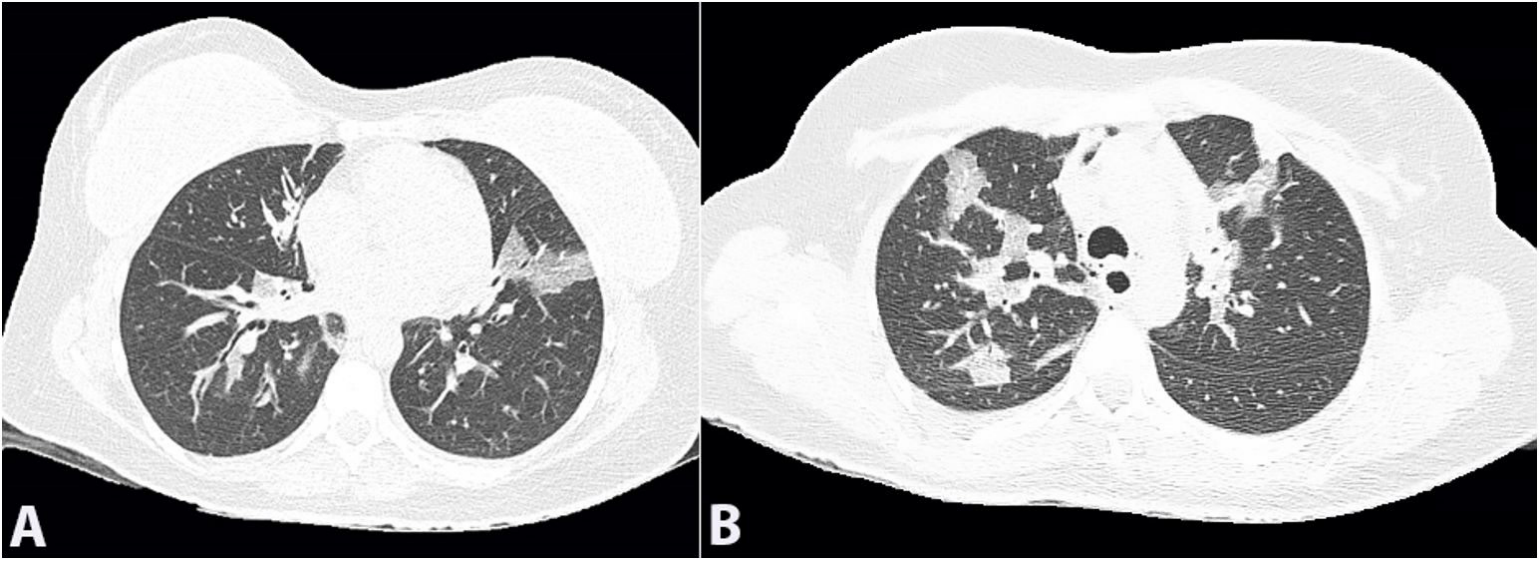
Chest CT images (lung window) in medium (A) and apical (B) axial planes show bilateral ground-glass opacities with consolidation areas involving all pulmonary lobes.
